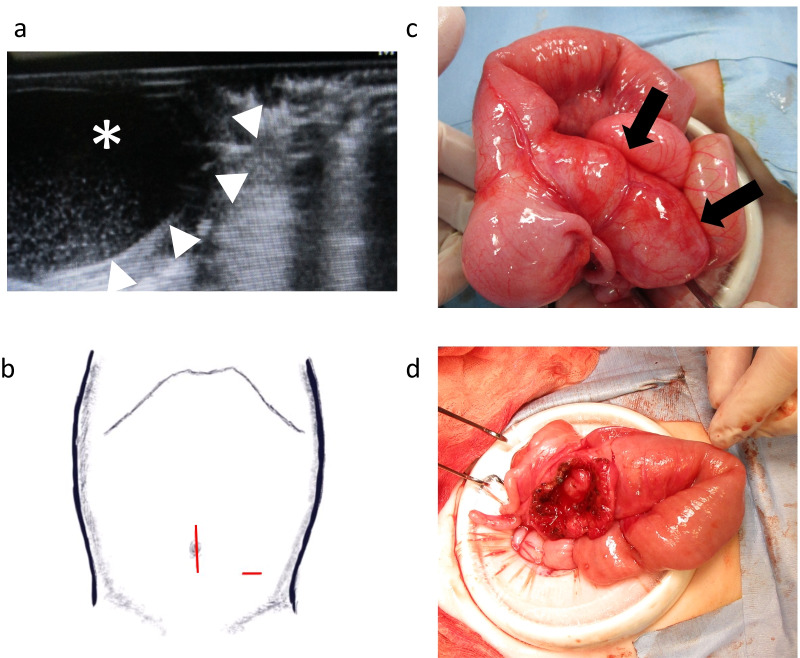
Operative findings in Case 2. a Intraoperative ultrasound of the duplication cyst, containing fluid (* and arrowheads). b Laparoscopic surgery was commenced with a midline incision at the umbilicus (25 mm in length). An additional 5 mm port was inserted in the left lower abdomen. The midline incision at the umbilicus was then extended to exteriorize the cyst. c Cystic mass in the terminal ileum (40 mm, arrows) was observed. We converted to open laparotomy when we detected and diagnosed the mass as a duplication cyst. d Mucosa of the cyst was dissected
